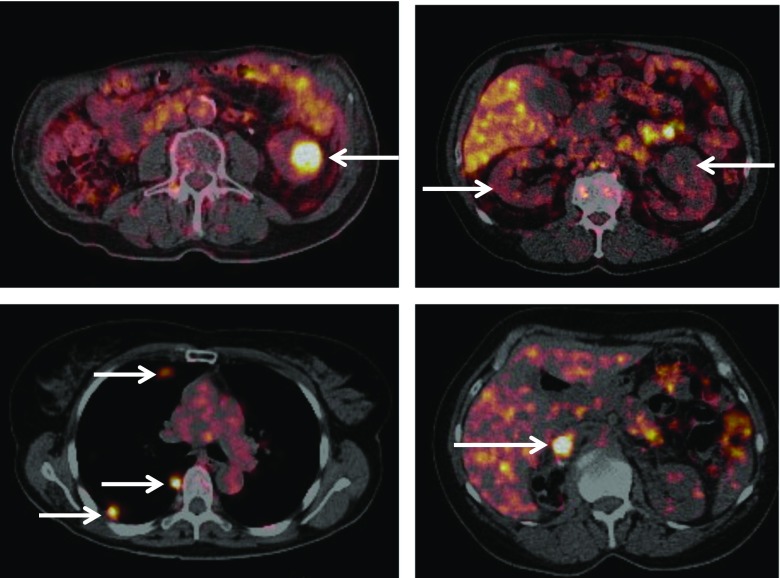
^89^Zr-Girentuximab PET imaging. *Top left*: A 76-year-old patient presenting with a Bosniak category 3 renal cyst in a solitary kidney and decreased renal function. ^89^Zr-Girentuximab PET/CT imaging indicated that it was a ccRCC, which was confirmed after partial nephrectomy. *Top right*: Patient presenting with bilateral growing renal masses. No uptake is seen in any of the lesions. Three biopsies confirmed that the masses were oncocytomas. *Bottom left*: Patient with a history of ccRCC who developed a large liver lesion and multiple pulmonary metastases. The ^89^Zr-girentuximab PET/CT image shows uptake in all lesions confirming metastatic ccRCC. *Bottom right*: Patient presenting with a 1-cm mass in the inferior caval vein 6 months after nephrectomy for ccRCC. The ^89^Zr-girentuximab PET/CT image shows high uptake in the mass and thrombectomy confirmed vital ccRCC
